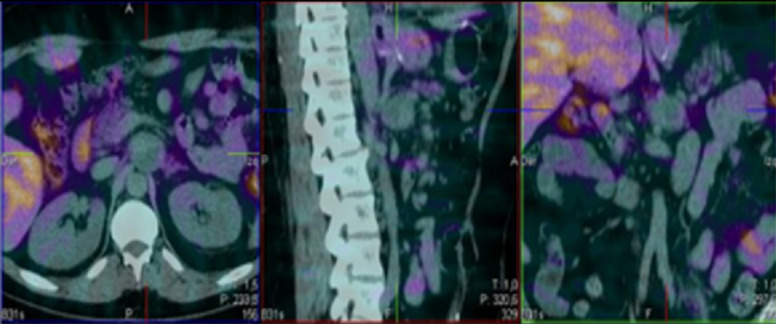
the (99m) Tc-octreotide Scintigraphy with SPECT/CT showed no evidence of pathological tumor uptake of the retroperitoneal mass
